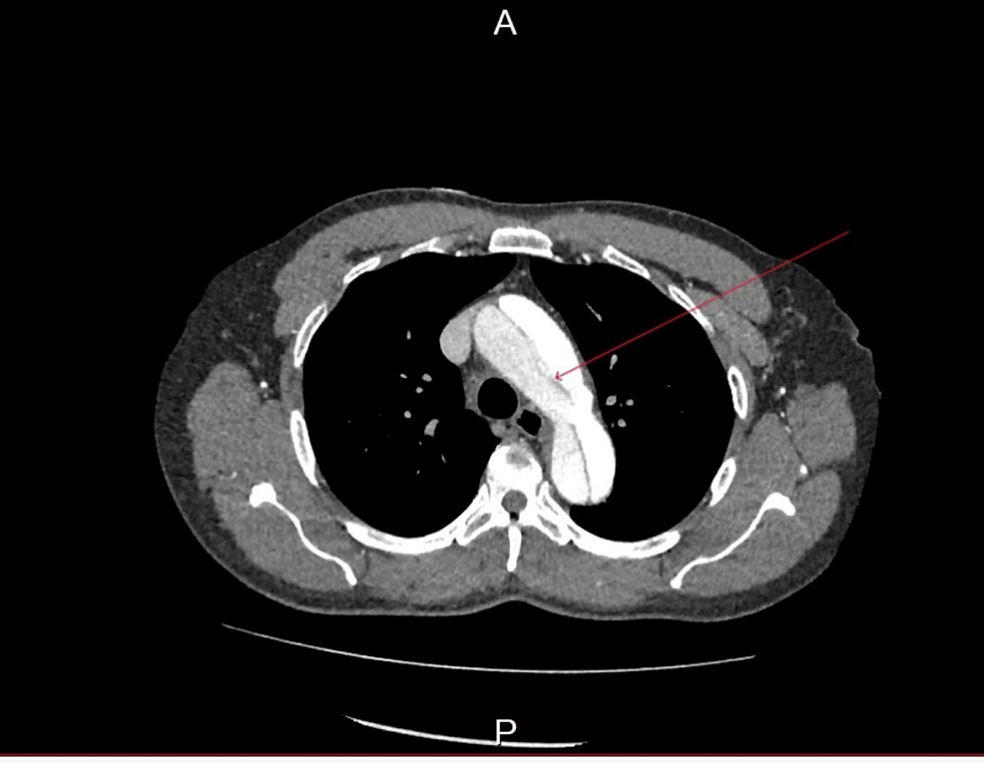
A CT angiogram of the aorta shows dissection of the arch of the aorta.
